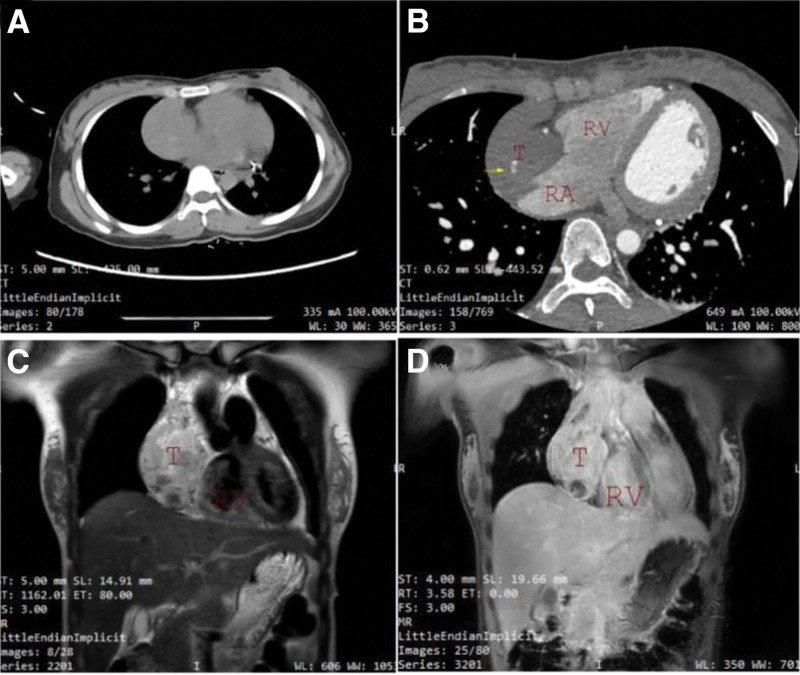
(A) Non-contrast CT (computed tomography) shows a large, slightly hyperdense, poorly homogeneous mass in the right atrium. (B) Contrast-enhanced CT reveals minimal contrast uptake (yellow arrow) with limited enhancement. (C) Non-contrast MRI demonstrates the mass broadly attached to the right atrial wall, showing a heterogeneous, slightly hyperintense signal on T2WI. (D) Postcontrast MRI shows marked heterogeneous enhancement with progressive contrast uptake and small patchy nonenhancing low-signal areas. CT = computed tomography, MRI = magnetic resonance imaging, RA = right atrium, RV = right ventricle, T = tumor.
